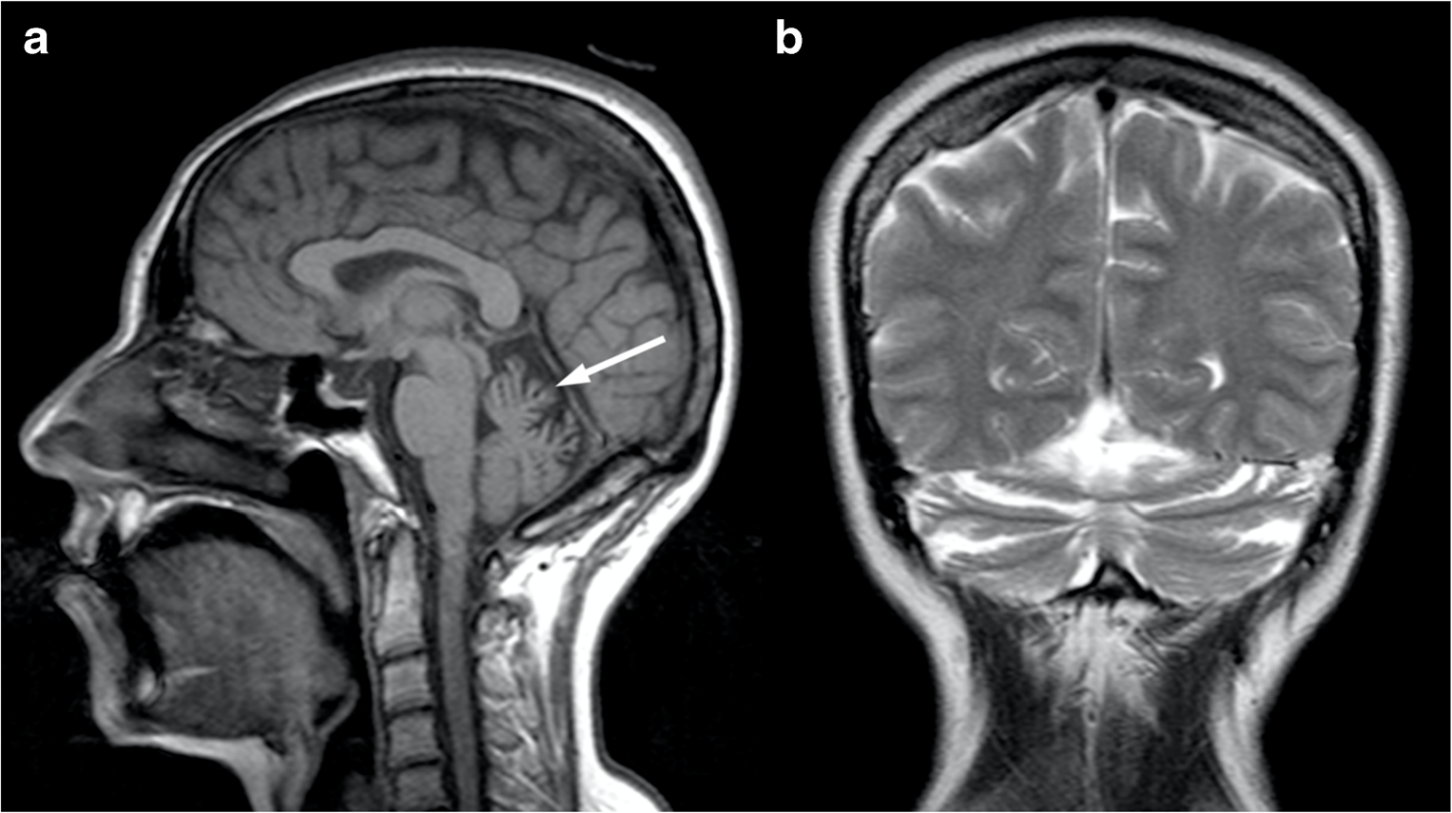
Brain MRI findings in a 56-year-old female SCA6. In the sagittal T1- (a) and coronal T2- (b) weighted images, it is appreciable a global cerebellar atrophy, with particular involvement of the vermis (arrow) and a relative sparing of the pons
